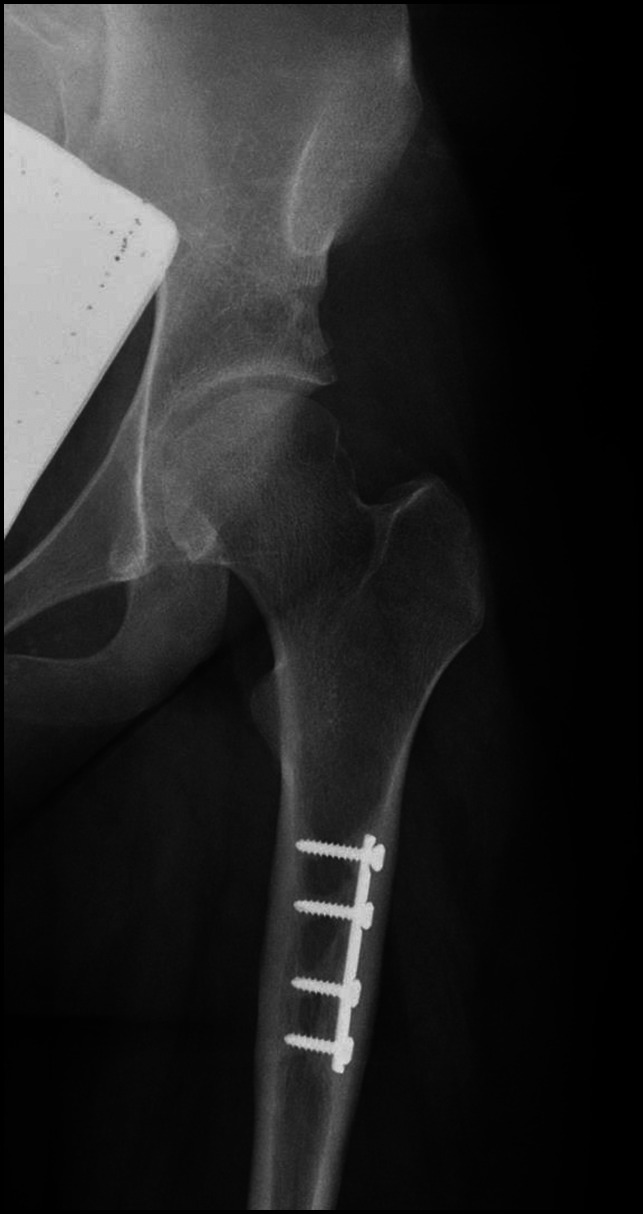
AP radiograph of the left hip of a 19-year-old female patient showing bone overgrowth of a retained plate.
